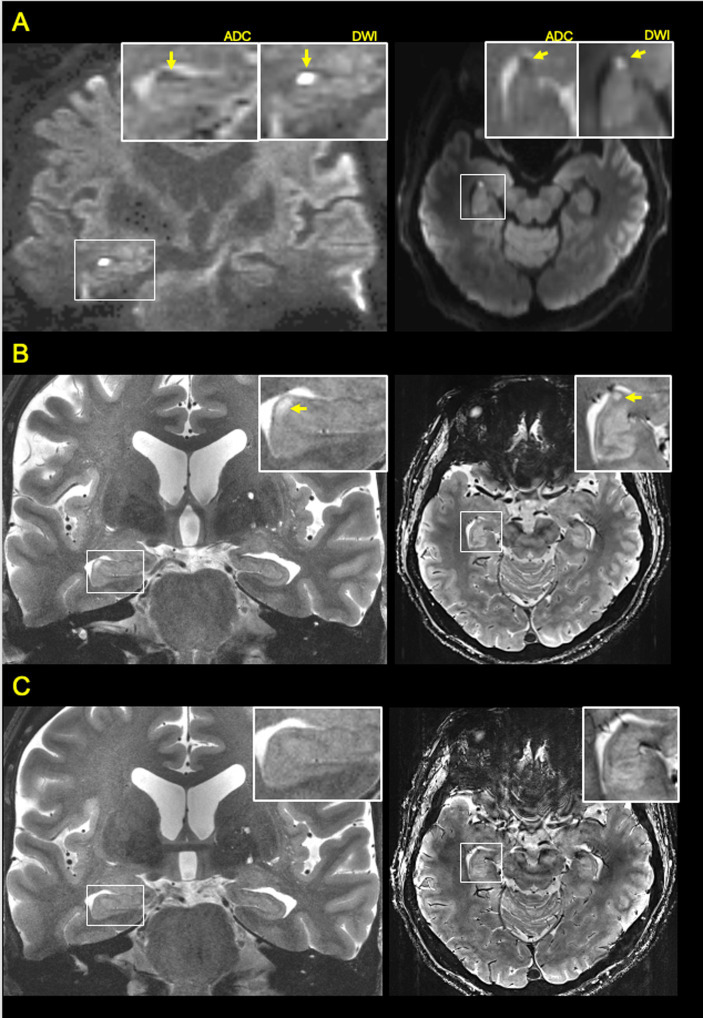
Serial 7 T MRI findings in Case 1. (A) Acute 7 T diffusion-weighted imaging (DWI) obtained 35 h after symptom onset demonstrates a focal punctate hyperintensity in the hippocampal CA1 region (arrow). Corresponding apparent diffusion coefficient (ADC) maps show focal hypointensity at the site of the DWI lesion, confirming restricted diffusion. Magnified views of the lesion are shown in the upper-right corner of each image; the left inset displays the ADC map, and the right inset displays the DWI image. (B) Corresponding 7 T T2-weighted image at the same time point shows a colocalized cortical-layer hyperintensity within CA1 (arrow), visible on both coronal and axial views. (C) Follow-up 7 T T2-weighted imaging performed on day 15 demonstrates complete resolution of the previously identified CA1 hyperintensity, with no residual signal abnormality.
